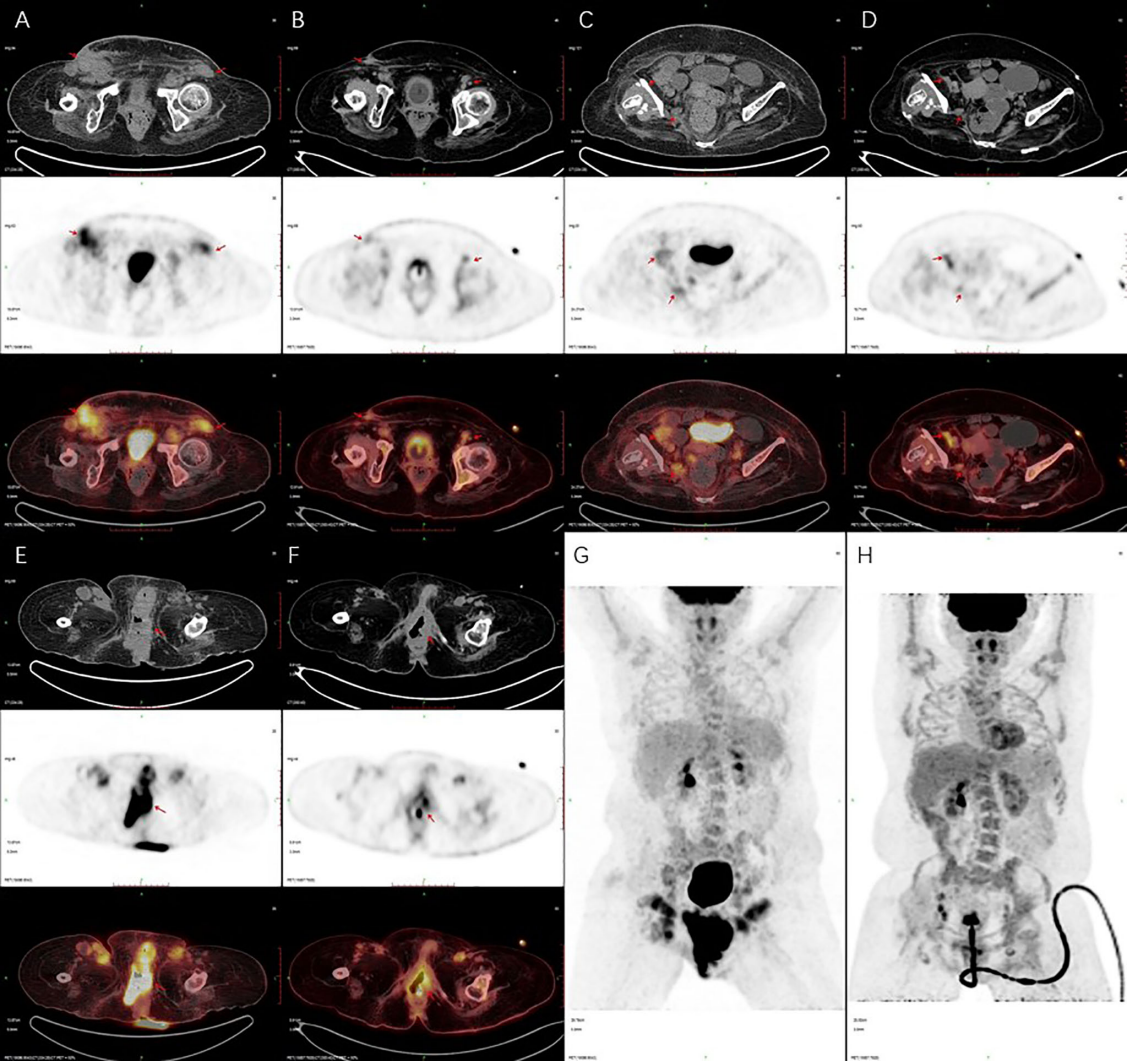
Positron emission tomography/computed tomography (PET/CT) imaging. PET/CT scan showed that metastatic lymph nodes were smaller and less than before (A–D), and the vulva lesions were smaller than before (E–H). (A, C, E, G) are PET/CT imaging taken in January 2019. (B, D, F, H) are PET/CT imaging taken in October 2019.
